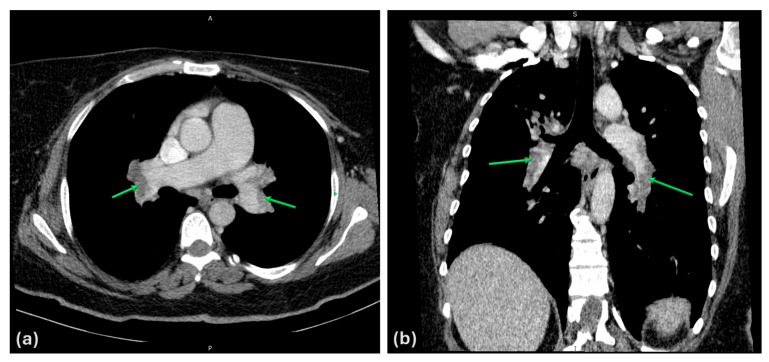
Chest CT angiography performed in 2017 shows acute massive pulmonary embolism (arrows) in the bilateral pulmonary arteries on axial (a) and coronal reconstructions (b).
